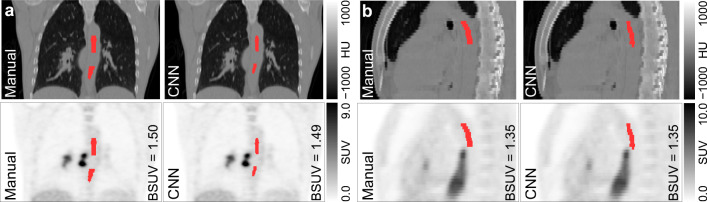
Comparison of manual and CNN-based aortic lumen delineation for two patients with a lung cancer (coronal view) and b esophageal cancer (sagittal view). CT (top) and corresponding PET (bottom) images are overlaid by the respective delineations (shown in red). Note that the CNN excludes the vicinity of the lesions from delineation in a way similar to the human observer
